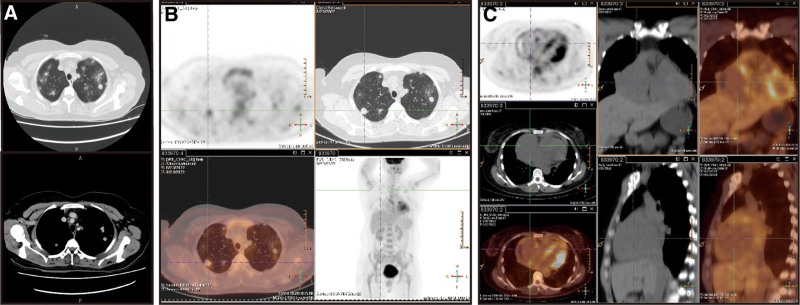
Image examination results. (A) CT showed multiple metastatic nodules in both lungs, with some nodules showing dot halo sign. (B) PET-CT suggested multiple solid nodules of varying sizes in both lungs, with increased glucose metabolism in some lesions, with a maximum SUV value of 3.2. (C) The right pericardium showed a soft tissue mass protruding into the pericardial cavity, with increased glucose metabolism at the margin and a maximum SUV value of about 4.7. CT = computed tomography, PET = positron emission tomography.
